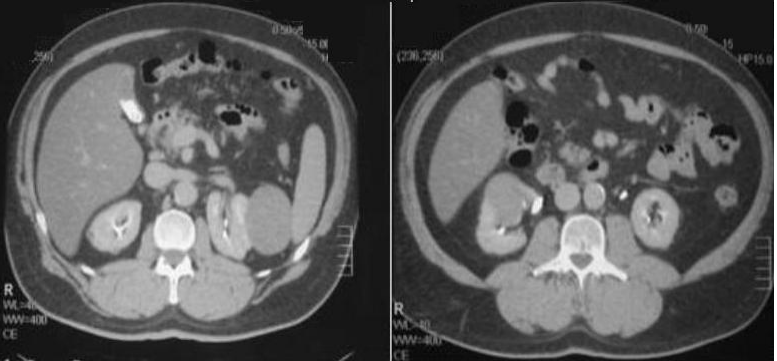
Abdomen: CT scan. Synchronous tumors of the kidneys.
